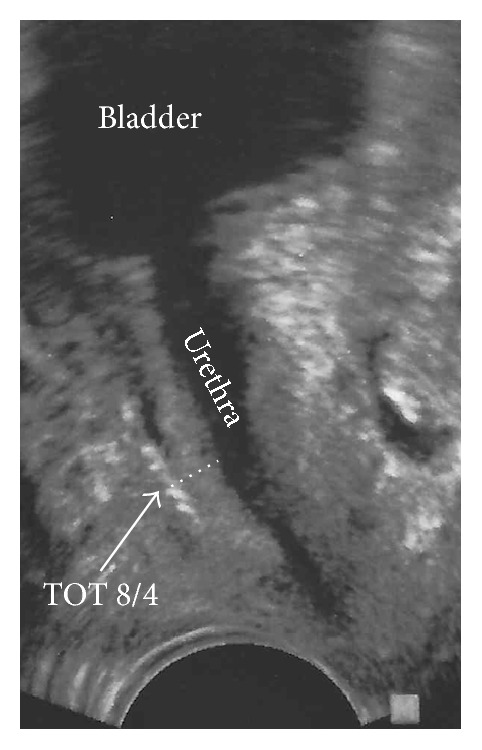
Transvaginal ultrasound in median sagittal view. A vaginal probe was used without exerting pressure to the midurethra. The center of the placed echogenic tape (TOT 8/4) is marked by the* white arrow head*. The shortest measured distance between the center of the tape and the lower part of the urethra is marked by the* broken line*.
